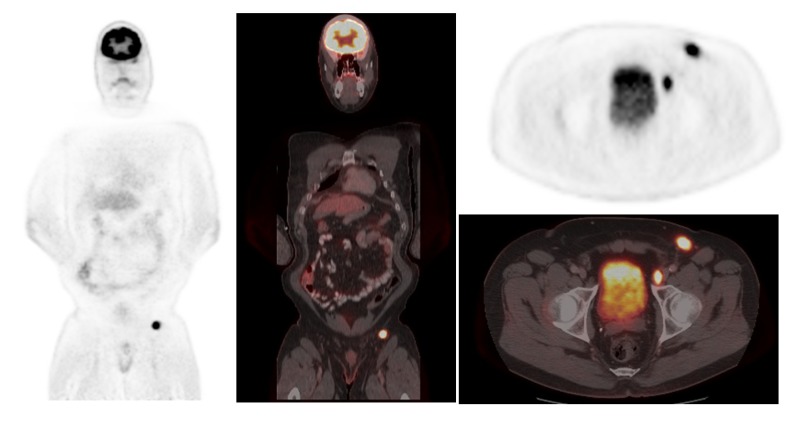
FDG PET/CT showing left inguinal node metastases in a patient with Merkel cell carcinoma.
